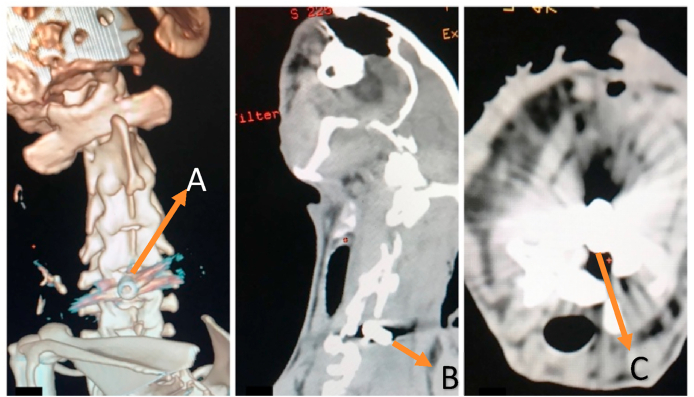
(A) 3D cervical spine shows screw in midline lamina C5 (B) CT Scan Cervical Spine Lateral view shows screw in spinal canal. (C) CT Scan cervical axial view shows screw in midline lamina C5, tip of screw in spinal canal.
